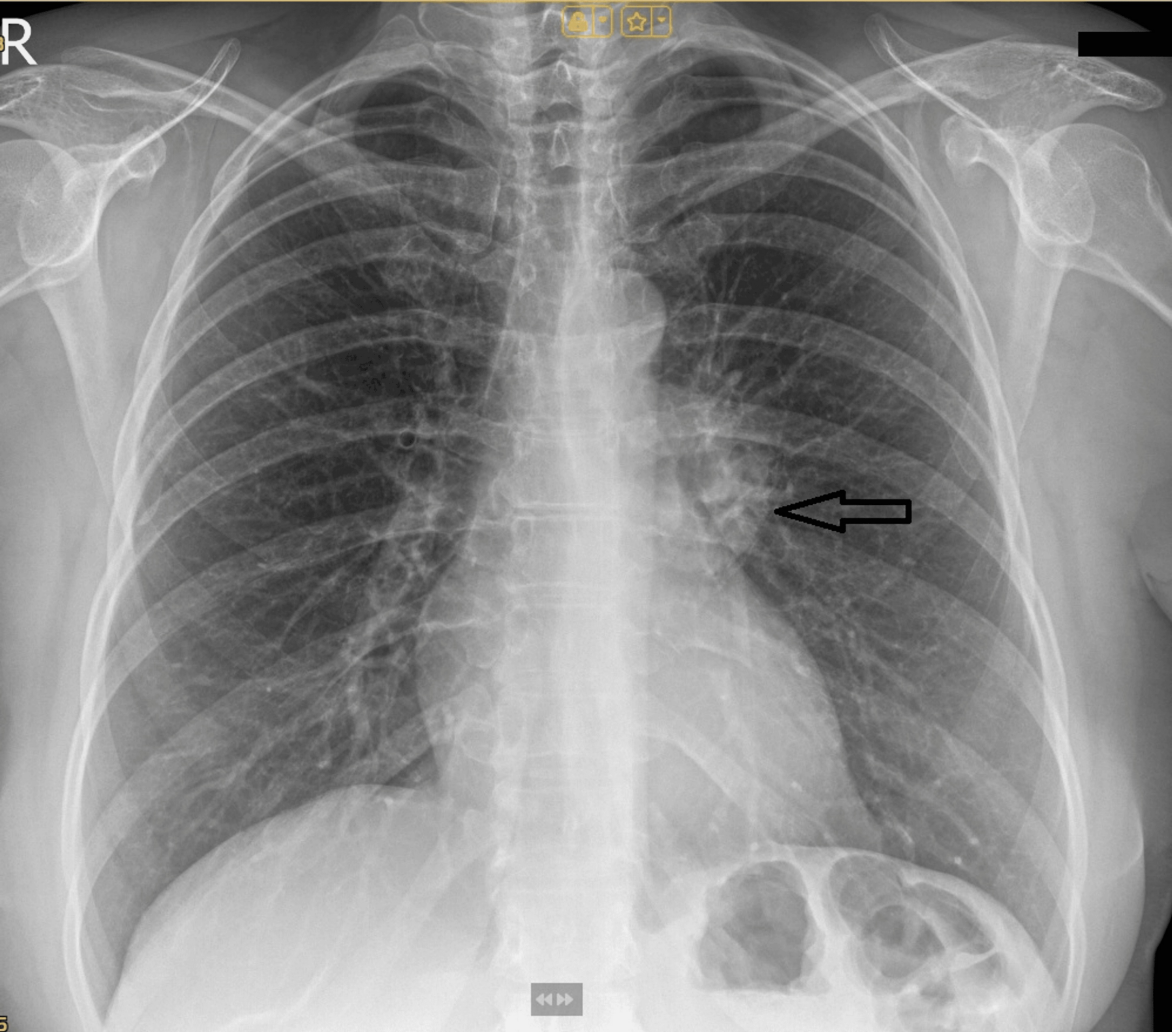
A preoperative chest radiograph (posteroanterior view) demonstrated a subtle, ill-defined left perihilar opacity (black arrow).
